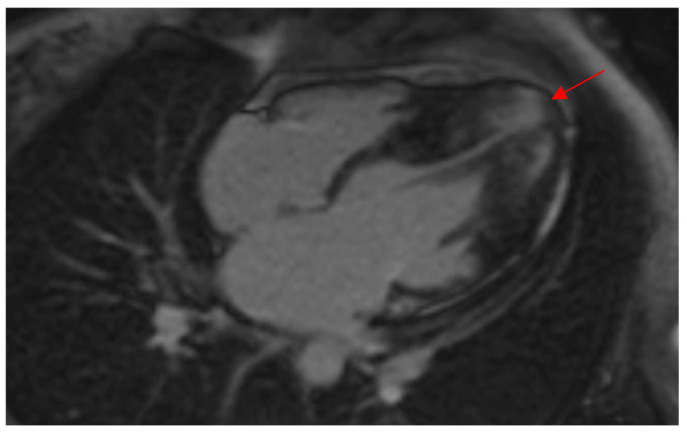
CMR demonstrates a thickened LV apex in a female patient of this study with wall thickness extending to the midventricular septum, indicating a “mixed ApHCM” (distal-dominant form). Note the presence of LGE in the hypertrophied apex (red arrow) as well as the apical aneurysm. ApHCM, apical hypertrophic cardiomyopathy; CMR, cardiac magnetic resonance imaging; LGE, late gadolinium enhancement; LV, left ventricular.
